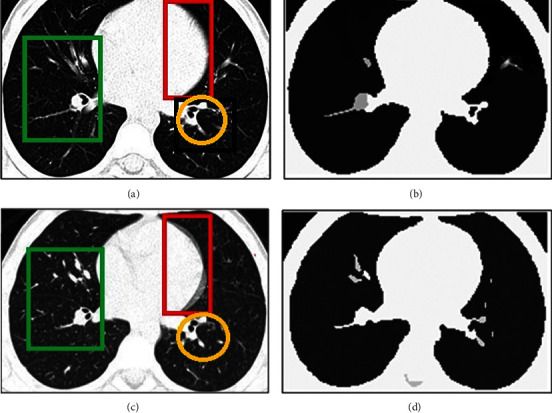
An impact of motion artefact on the segmentation performance for volumetric HRCT images with and without motion artefacts [6]. (a) Original VHRCT image, (b) Segmented original with superpixel. (c) Motion artifact blured VHRCT, (d) Segmented VHRCT with superpixel.
